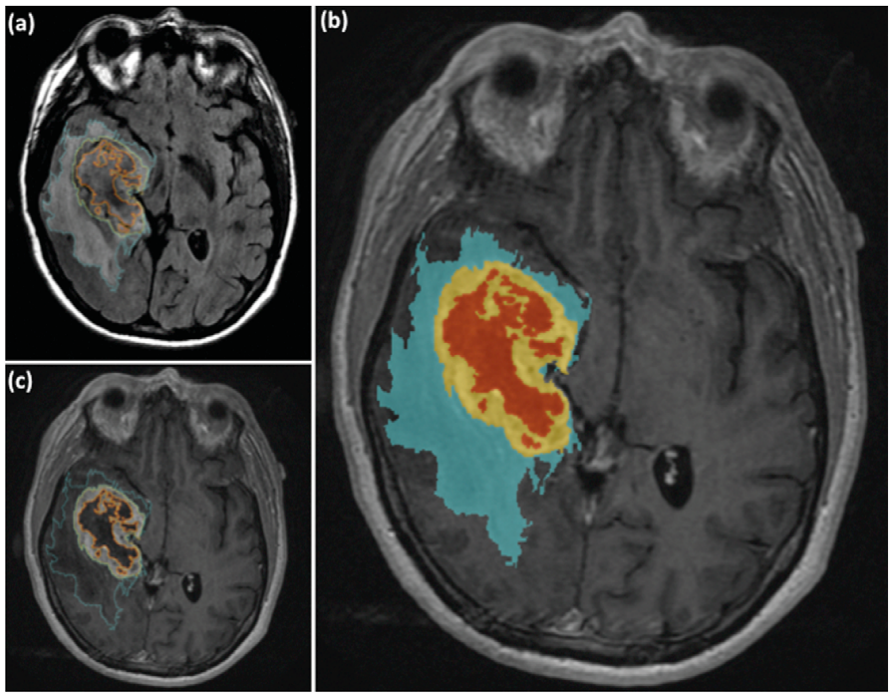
Volumetric brain tumor mapping of FLAIR, Necrosis, and Contrast Enhancement. A 55 year old male patient with a right temporal GBM. (a) Axial FLAIR image demonstrates segmentation (in blue) of the region of FLAIR hyperintensity corresponding to the area of edema/tumor infiltration. Notice the segmented enhancement (yellow) and necrosis (orange) that has been segmented on the T1WI post- contrast. (b) The segmented edema/tumor infiltration (blue), enhancement (yellow) and necrosis (orange) are seen overlaid on a base post- contrast T1WI. (c) Axial post-contrast enhanced T1WI demonstrates the segmentation of the enhancement (yellow) and necrosis (orange).
